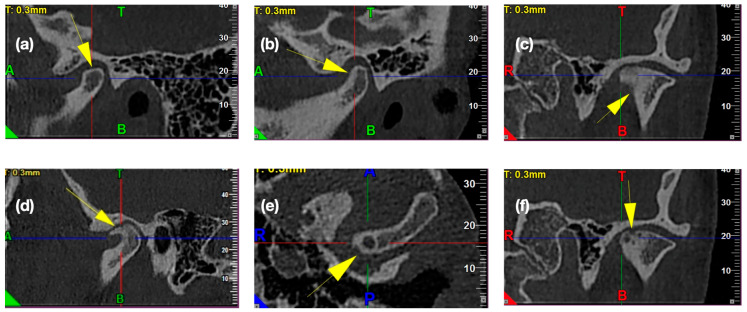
Osteoarthritic changes in the temporomandibular joints’ CBCT images: (a) Articular surface flattening, (b) subcortical sclerosis, (c) generalized sclerosis, (d) osteophyte, (e) subcortical cyst, and (f) erosion.
